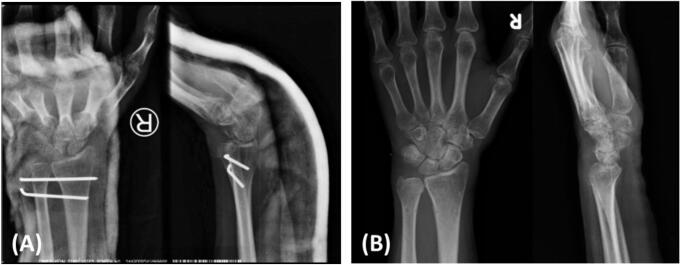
Postoperative wrist radiograph (A) revealed that DRUJ complex was held in place by the K-wire. Radiograph at 4th months (B) revealed an intact DRUJ complex and union of ulnar styloid fragment.
